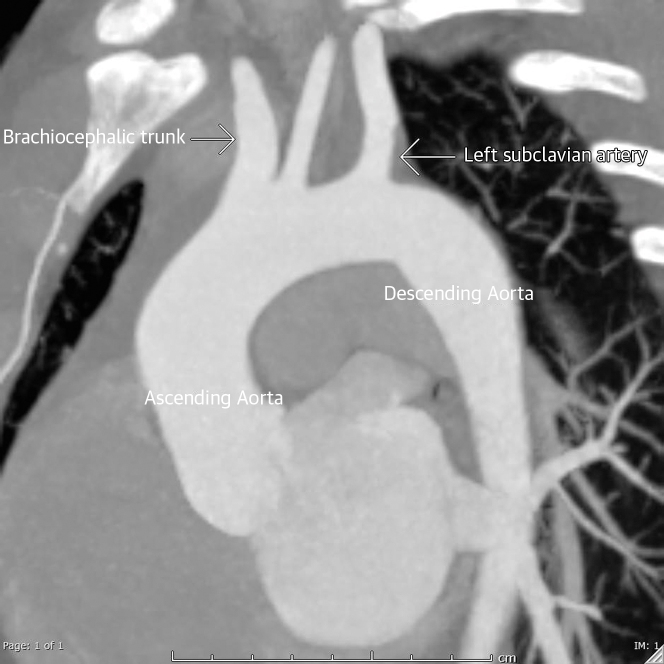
Computed Tomography Aortic Angiogram Findings were consistent with large vessel vasculitis, mainly Takayasu arteritis. Oblique sagittal maximum intensity projection image of the aortic arch showed focal eccentric mural thickening of the proximal left subclavian artery causing mild stenosis.
